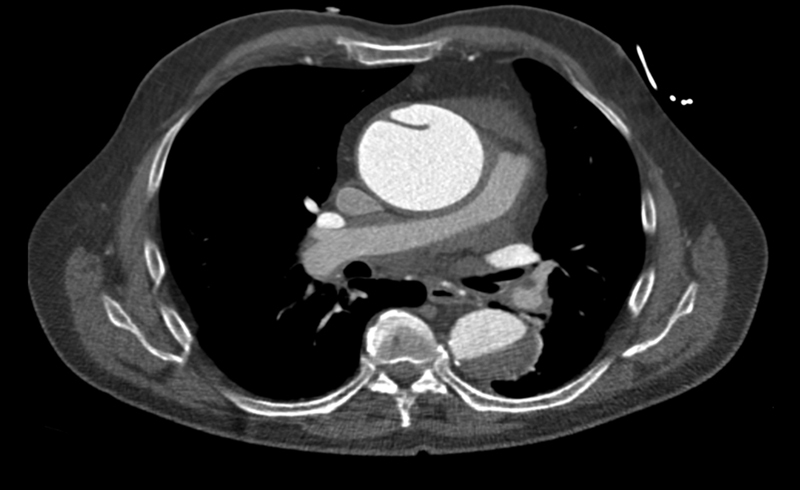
Computed tomography aortogram confirming the diagnosis of acute type A aortic dissection.
